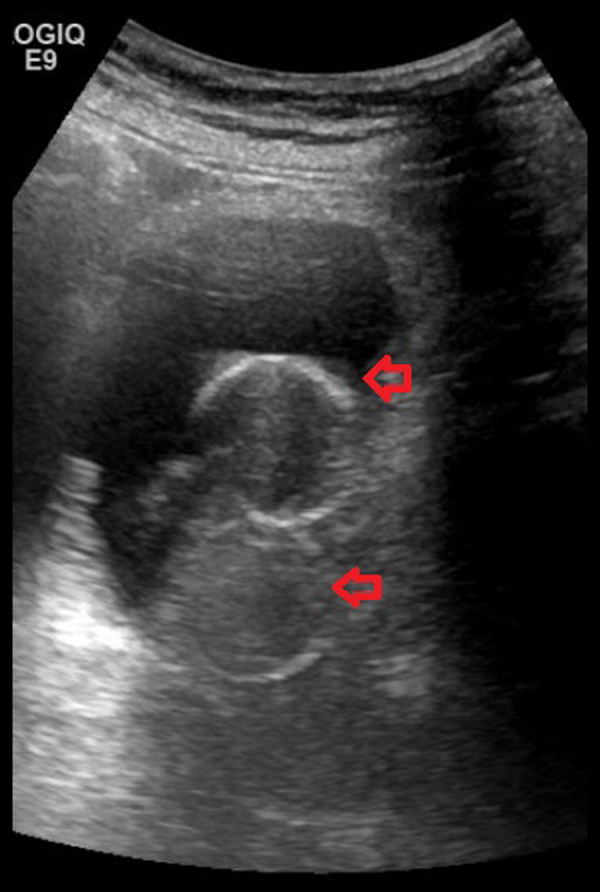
Ultrasound scan of urinary bladder performed in August 2015 (eight years after spinal injury) showed presence of both urethral catheter balloon (bottom arrow) and suprapubic catheter balloon (top arrow) within the small capacity urinary bladder
